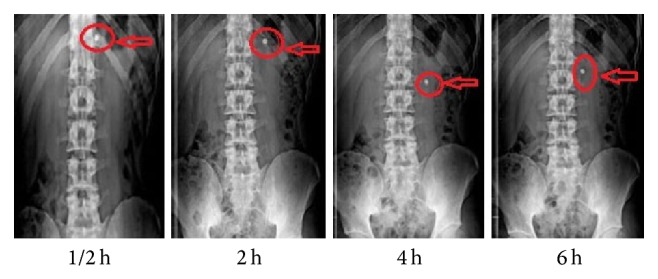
X-ray graph of optimized batch F8 (polyox WSR205 and xanthan gum).
